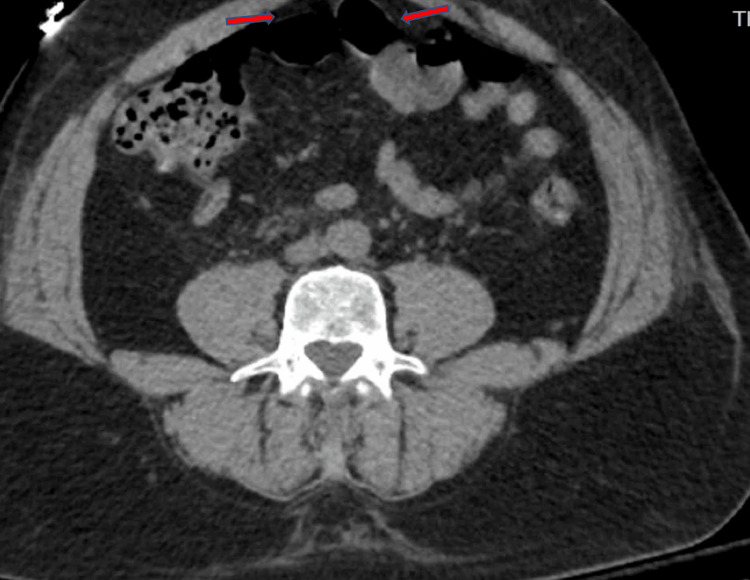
Computed tomography of the abdomen and pelvis. Axial image at the umbilical level showing a tiny fat-containing paraumbilical hernia with adjacent skin thickening and inflammation (arrows).
